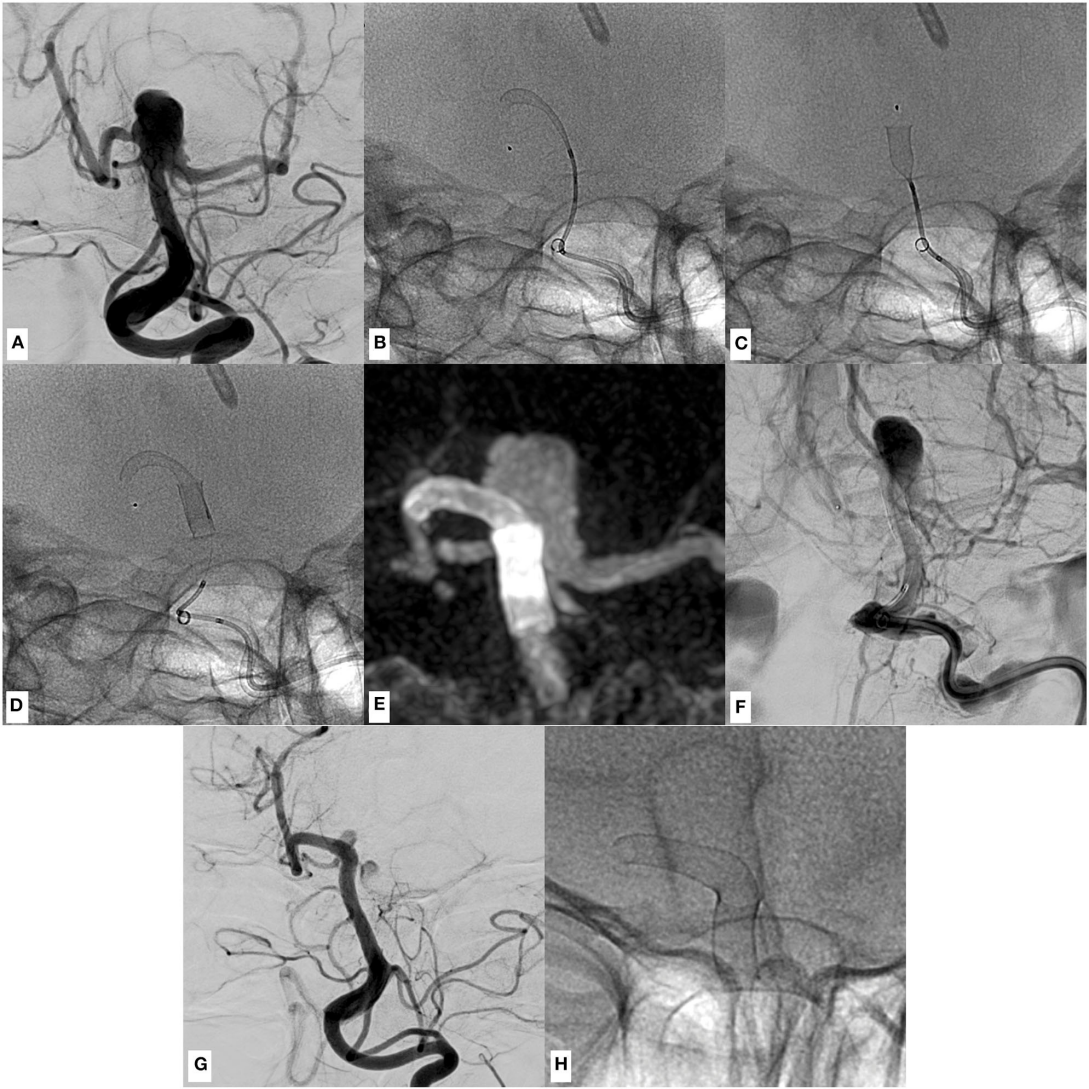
An example of p48MW hydrophilic coating (HPC) implantation in the right posterior cerebral–basilar artery (BA) junction for treatment of a broad-based, partially thrombosed, un-ruptured aneurysm arising from the basilar tip, causing progressive hydrocephalus. (A) Conventional angiogram in working projection shows the perfused part of the saccular, broad-based aneurysm of the BA–posterior cerebral artery (PCA) complex (neck, 7 mm; fundus, 7 × 8 mm). (B) Positioning of the first p48MW HPC. The distal landing zone, aiming to cover the smallest perforator-rich area as possible, was defined as comparatively short. Note the distal end of the movable wire (radiopaque olive) within the distal P1 segment, which was used to further stabilize the system during implantation. In retrospect, a more distal positioning of the MW in the middle part of the P2 segment may have prevented dislocation of the first device, as demonstrated in the following images. (C) Simultaneous to the deployment of the device, significant distal shortening and subsequential proximal dislocation of the entire device occurred, requiring the implantation of a second p48MW HPC in order to sufficiently cover the broad aneurysmal orifice. (D) Device radiogram showing the second p48 implanted in telescoping technique. Now the aneurysm is covered sufficiently. Initially, wall apposition/flow diverter opening appeared to be only moderate, which resolved a few minutes after implantation without the necessity for further manipulation. (E) Maximum intensity projection of the flat-panel CT shows the flow diverter stent (FDS) construct and its relation to the BA–PCA junction and the broad-based aneurysm. (F) Venous phase of a digital subtraction angiography (DSA) run in working projection after successful implantation shows protracted opacification of the aneurysm sac (O'Kelly Marotta: A3) indicating the extent of the flow diversion effect. (G) Follow-up DSA in working projection 3 months later shows the remodeled posterior circulation with a minimally perfused remnant of the aneurysm. (H) Device radiography in corresponding projection.
